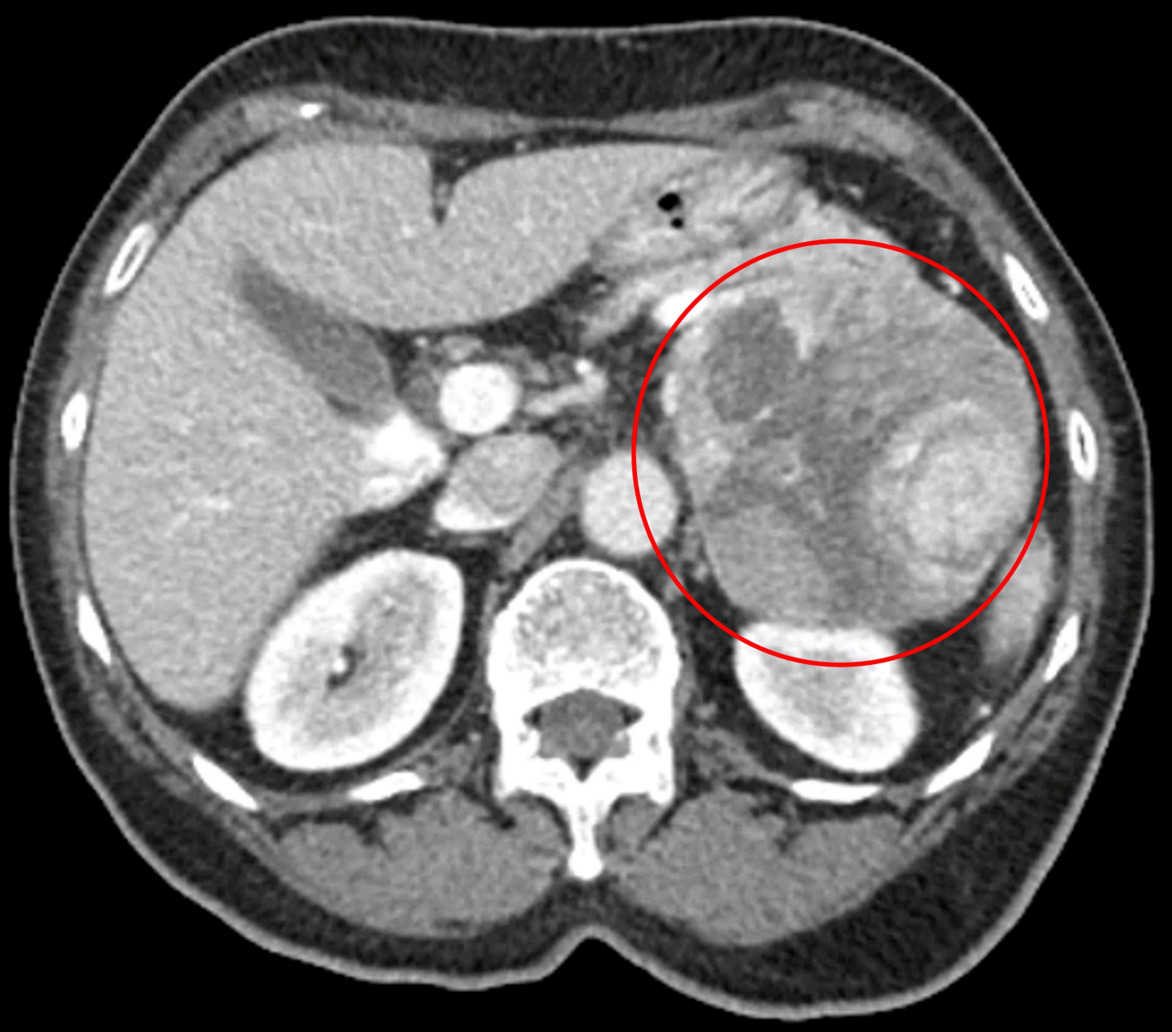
Axial abdominal computed tomography scan showing a 113 mm left adrenal mass (circled)
